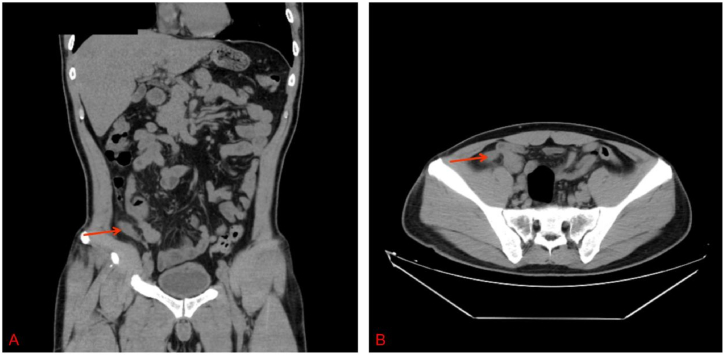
(A) A cross-sectional CT image revealing appendicitis. (B) A coronal section CT image showing appendicitis. CT, computed tomography.
